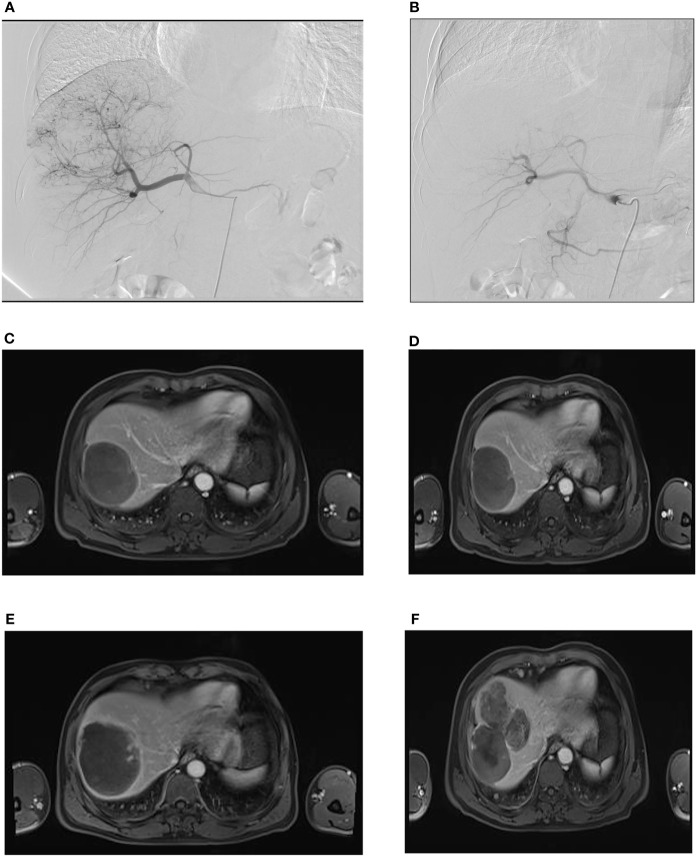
Rough-type tumor margins observed on an axial MR image. A male patient, aged 55 years, was admitted to our hospital with a complaint of right upper abdominal pain for 3 days. (A) During the first administration of DEB-TACE, hepatic arteriography showed massive abnormal hyperchromatism in the right posterior lobe of the liver. (B) Abnormal hyperchromatism disappeared at the end of the arterial phase, as observed through contrast reexamination after DEB-TACE; (C) the patient was included in the follow-up after the second administration of DEB-TACE, and MR images showed a rough-type margin on the left side of the tumor (black arrow). (D) One month after the start of the follow-up period, the rough-type margin (black arrow) was slightly larger than that in (C). (E) Three months after the start of the follow-up, the thickness of the rough-type margin (black arrow) increased and invaded the surrounding area (white arrow). (F) Six months after the start of the follow-up period, the tumor completely recurred in the corresponding direction (white arrow, black arrow) of the rough-type margin in (E).
